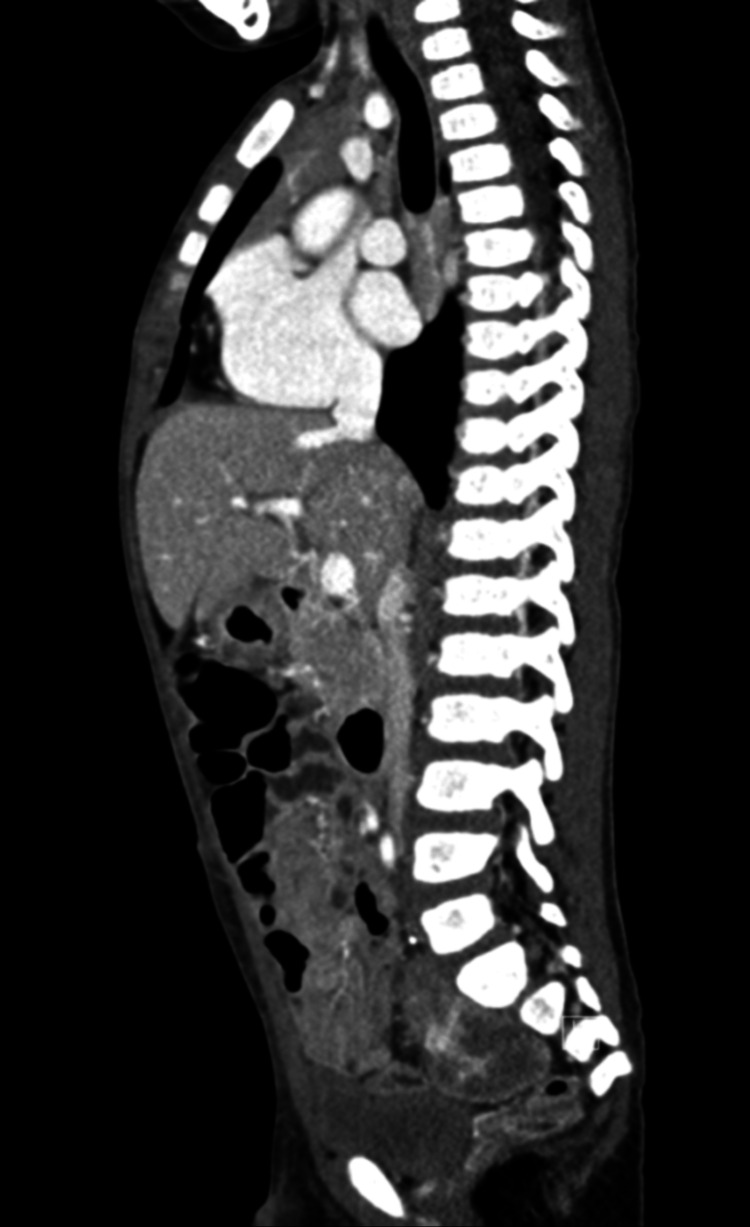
A sagittal image of the CT scan revealed a well-defined, hypodense, lobulated, heterogeneously enhancing, soft tissue mass in the pelvis.
